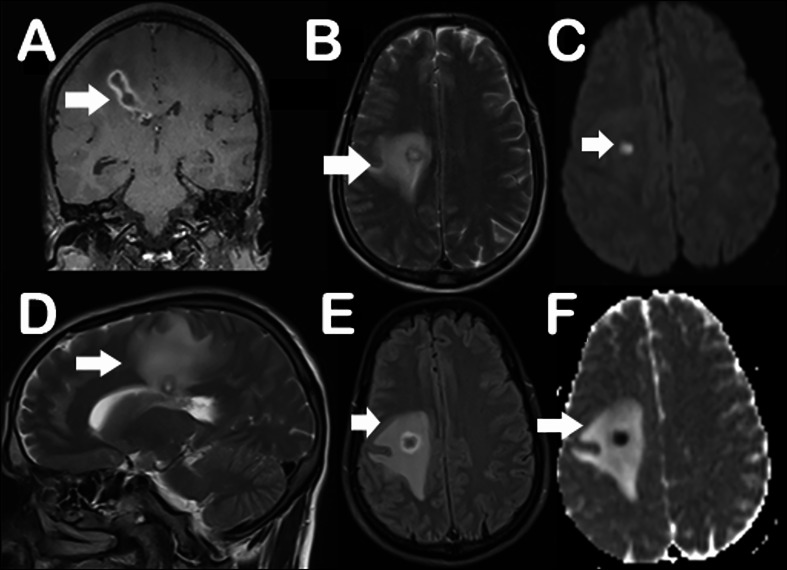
MRI images of 38-year-old male with Neuromelioidosis. Coronal MPRAGE sequence of MRI brain shows an irregular lesion with peripheral hyperintense signal in the right frontal cortex (arrow in A). T2 axial, sagittal and FLAIR sequences showing hyperintense peripherally enhancing lesion extending along the white matter to the body of corpus callosum (arrows in B, D-E). ADC and DWE axial images showing central diffusion restriction in the corresponding lesion (arrows in C, F)
